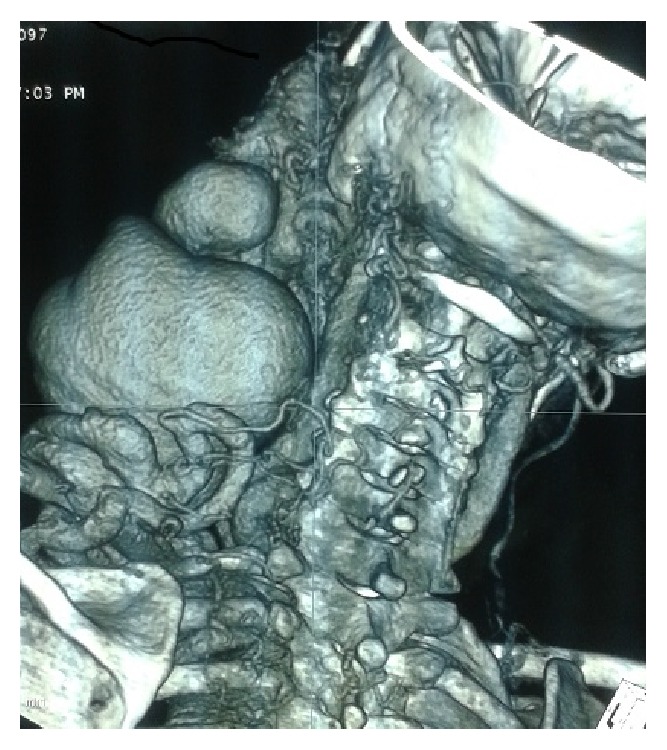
CT scan. Posterior view of cervical AVM showing connections with vertebral.
